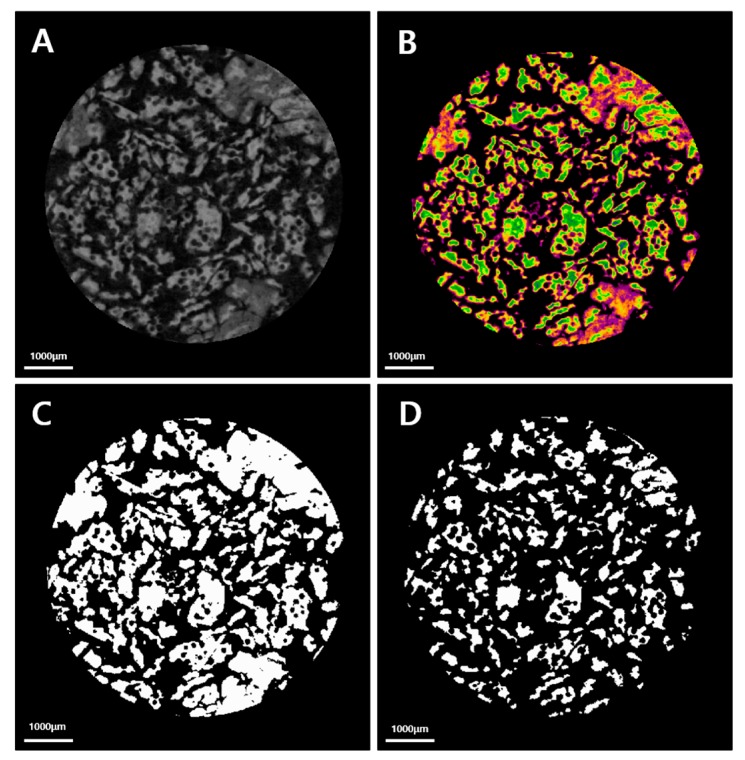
Micro-computed tomographic (CT) images of regions of interest. (A) Reconstructed image; (B) Color image (yellow and green-bone graft material and orange and purple-new bone); (C) Total bone (bone graft material and new bone) image; (D) Bone graft material image.
